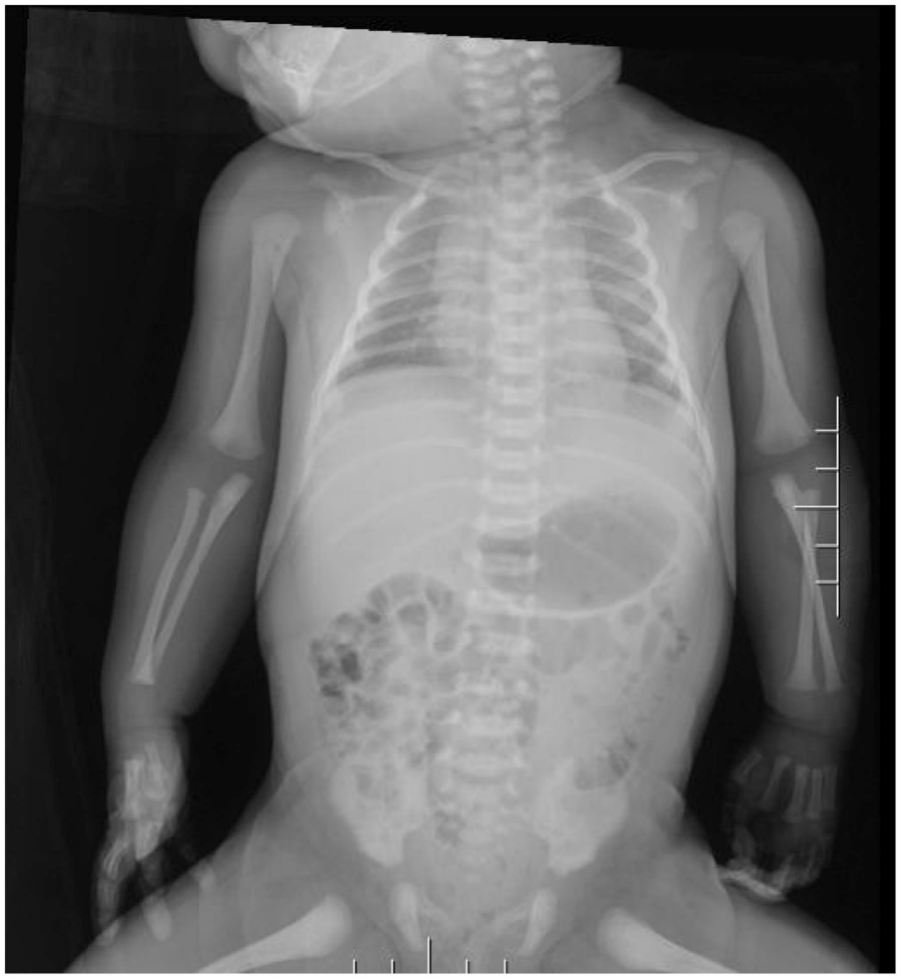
Chest radiograph. Chest radiograph demonstrates increased lung markings, particularly in bilateral lung fields. Cardiac silhouette appears within normal limits for age. No focal consolidation, pleural effusion, or pneumothorax is identified.
